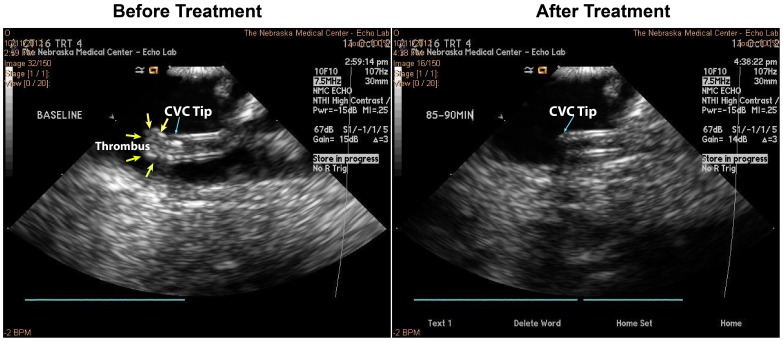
Intracardiac echocardiography from a case of longer treatment duration. Images before and after longer duration (90-minute) ultrasound and micobubble treatment in a case that showed complete thrombus dissolution.
